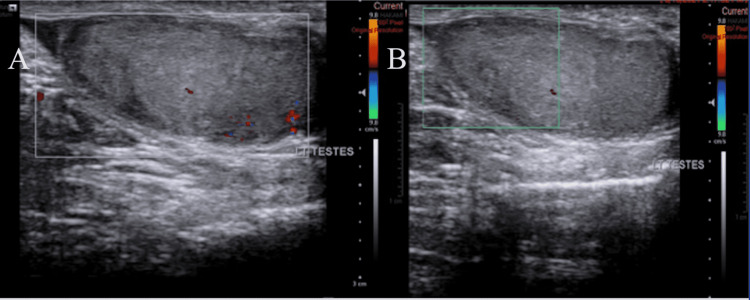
Initial color Doppler ultrasound of the left testis that showed a hypoechoic area with an absent color flow in that area
